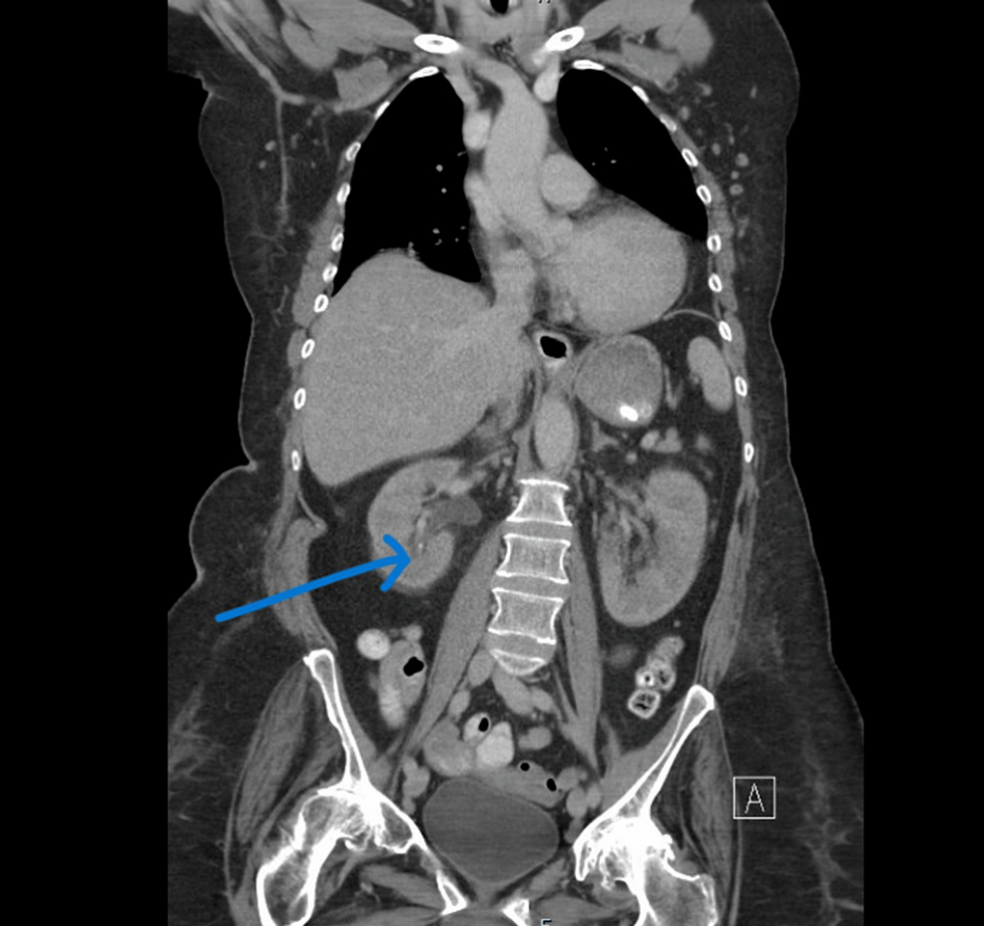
Coronal view of the 4mm non-obstructing stone in the inferior collecting system of the right kidney on the CT scan
